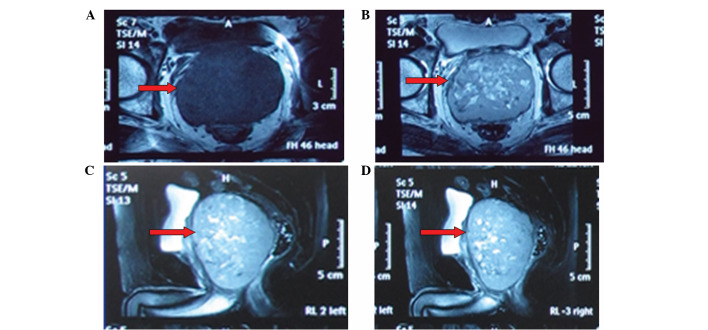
(A) Tumor appeared slightly hypointensive on the axial, unenhanced, T1-weighted magnetic resonance imaging (MRI). (B) Tumor appeared hyperintensive with internal irregular fluid-intense areas on the axial, unenhanced, T2-weighted MRI. (C and D) Tumor was predominantly confined to the prostate without evidence of direct involvement by the adjacent organs. The arrows indicate the tumor.
